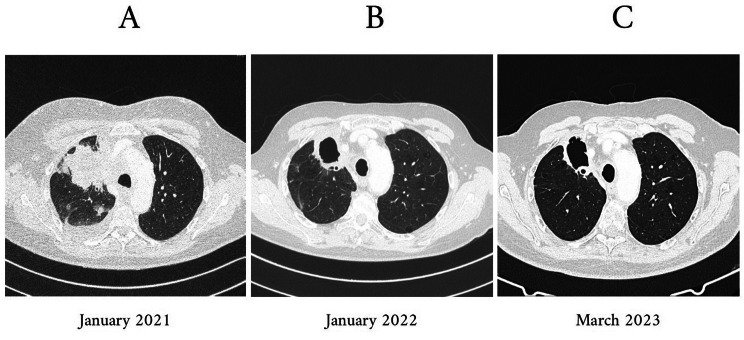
Chest CT images performed (A) during the patient’s diagnosis of cancer in January 2021, (B) 1 year later, in January 2022, before starting NTM-LD treatment and approximately three months after discontinuation of durvalumab therapy, and (C) in March 2023, thirteenth month after the start of NTM-LD treatment and five months off immunomodulatory therapy with ICI.
